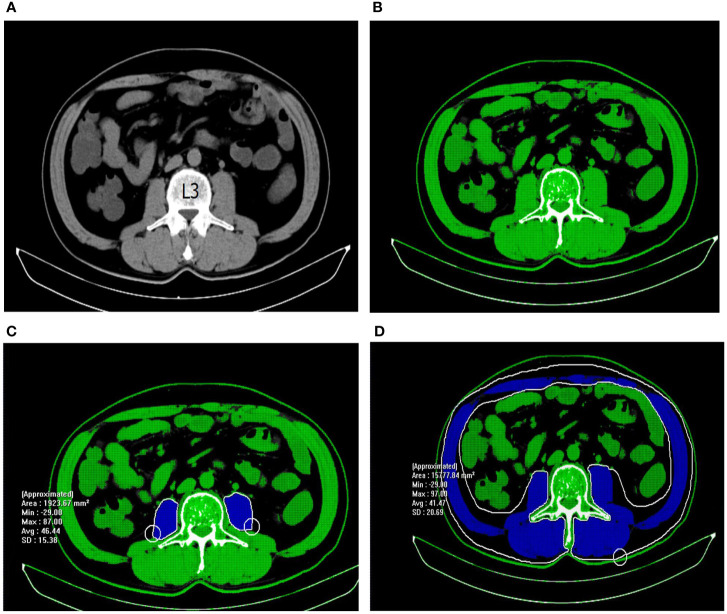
Measurement of abdominal muscle density and area with abdominal non-contrast CT images. (A) The third lumbar vertebra was chosen as a landmark. (B) Tissues with HU thresholds of − 29 to 150 are automatically outlined in green. (C) The selected region of psoas muscle was manually outlined in blue. PMD = 46.44 HU; PMA = 1923.67 mm^2^. (D) The selected region of total abdominal muscle was manually outlined in blue. TAMA=15777.84 mm^2^.
